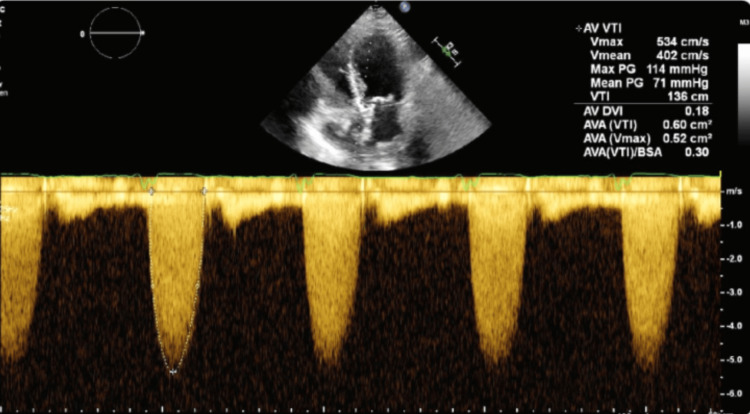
Transthoracic echocardiogram showing velocity across the bioprosthetic valve of 5.4 m/s, a mean gradient of 71 mmHg, and an aortic valve area of 0.5 cm.
